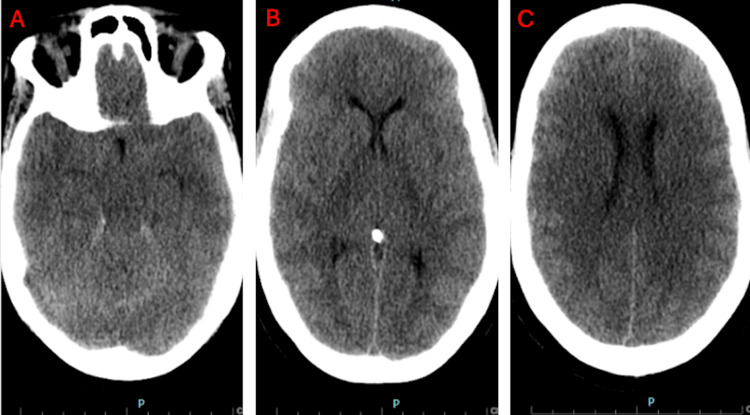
Axial CT scan of the head without contrast. (A-C) Caudal to cranial sequence demonstrating diffuse brain edema.
